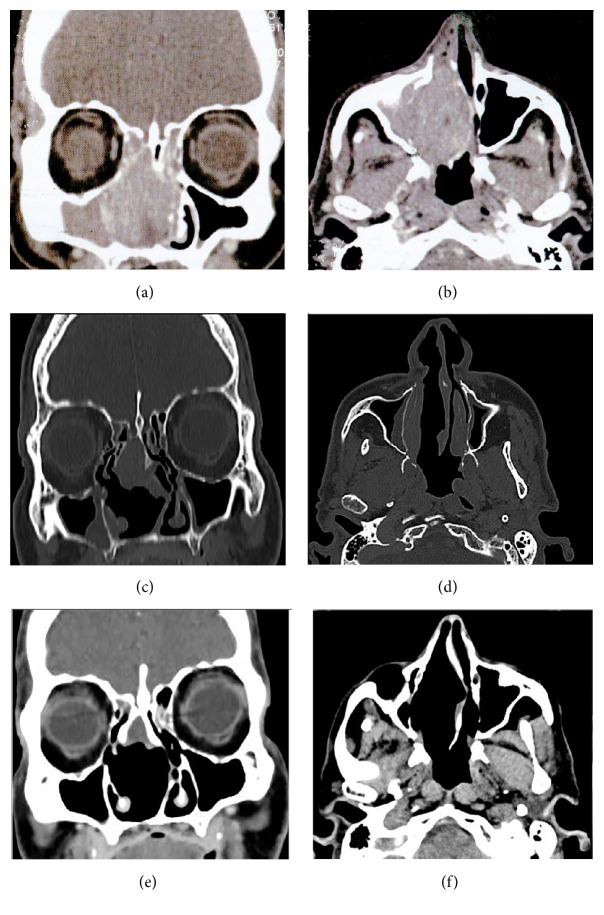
(a) and (b): pretreatment: computed tomography of the facial sinuses. Presence of a solid voluminous mass extending throughout the right nasal cavity, measuring 3.8 × 3.1 × 5.1 cm along its longest axes. This mass is infiltrating the left nasal cavity, ethmoidal cells, and cribriform plate, causing erosion to the plate. (a) Coronal plane. (b) Axial plane. (c) and (d): midway through treatment: computed tomography of the facial sinuses. (c) Presence of a solid lesion affecting the ethmoidal cells, nasal cavity, and right sphenoid sinus, with the tumour extending as far as the floor of the sella turcica on the right, with erosion. No signs of pituitary involvement. Widening of the sphenoethmoidal recess due to infiltration of the tumour mass. Thickening of the mucosa occupying the maxillary sinuses bilaterally, principally on the right (coronal plane). (d) Lesion lodged in the right nasal cavity with deviation of the septum to the left (axial plane). (e) and (f): following treatment: computed tomography of the facial sinuses. Improvement of the sphenoid and right maxillary sinuses, with almost complete resolution of the lesions seen in previous exams. (e) Coronal plane. (f) Axial plane.
